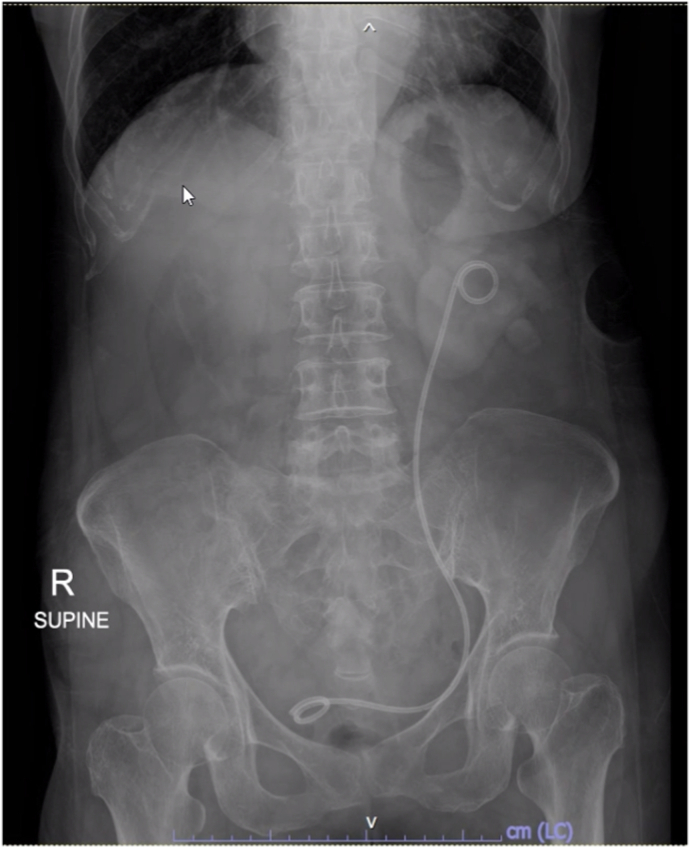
Postoperative abdominal radiograph showing complete stone clearance.
